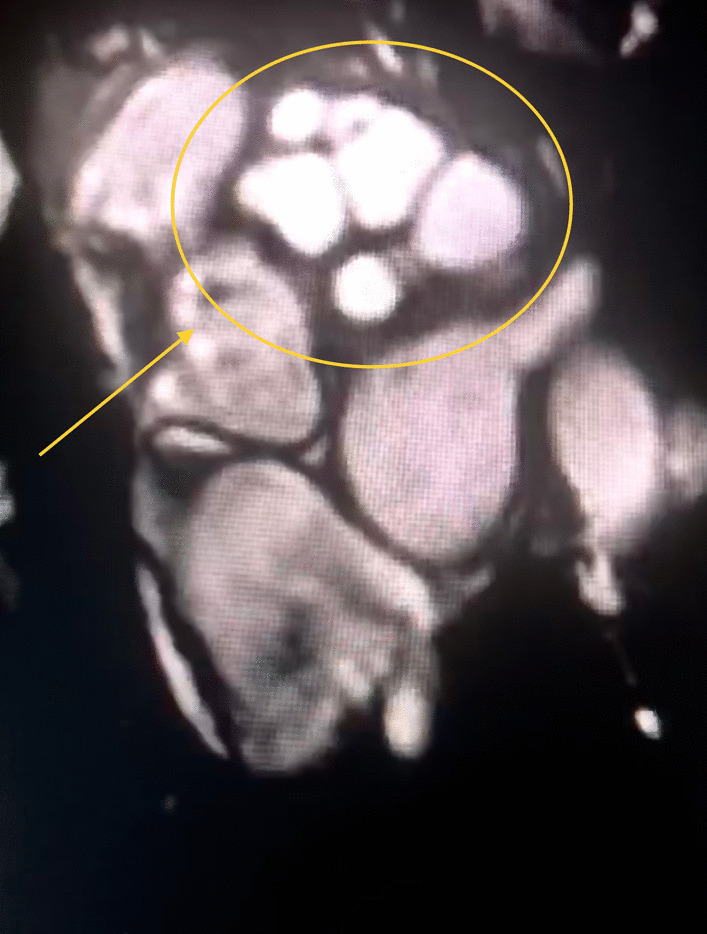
Hypointense multicameral cystic mass on MRI
